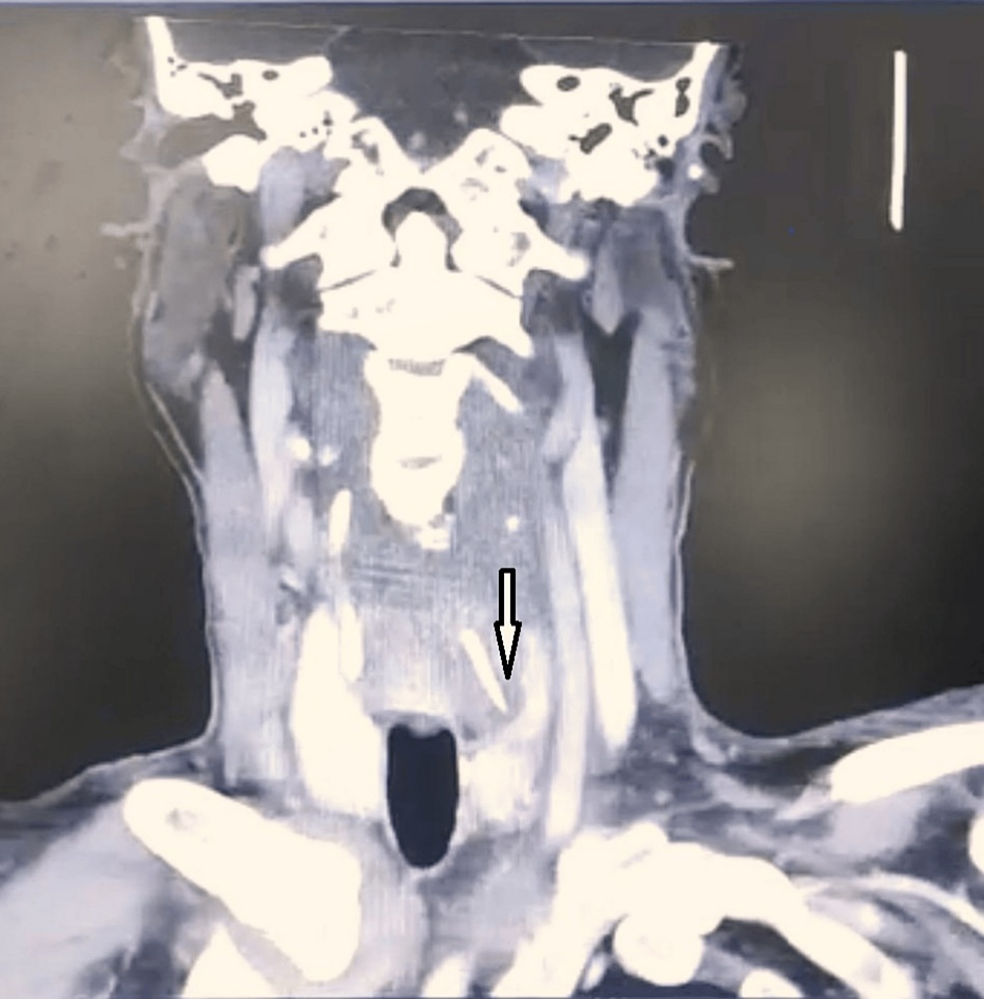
CT of the neck (coronal cut contrast-enhanced) showing the location of the fish bone over the left superior horn of the thyroid cartilage.
